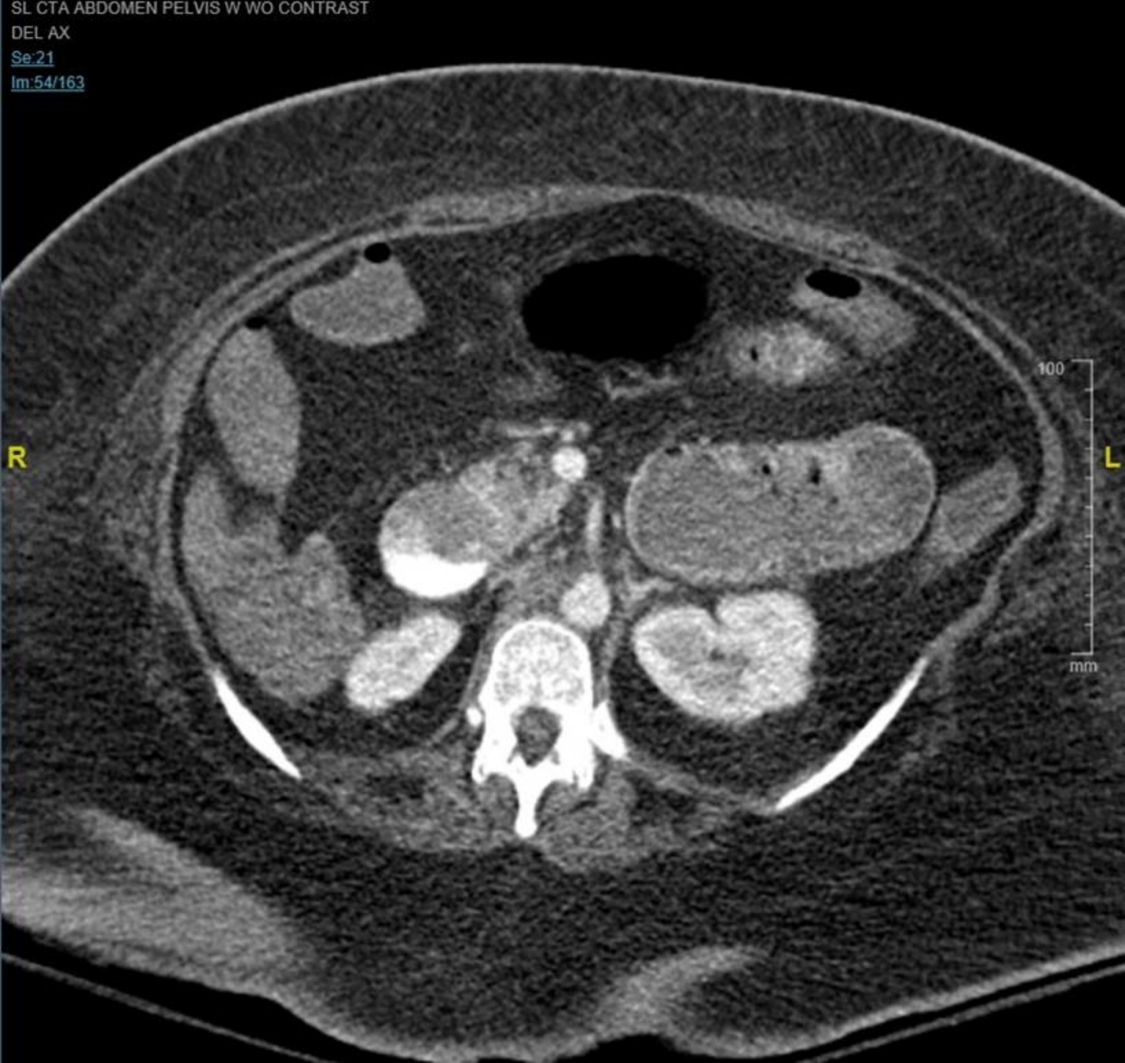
CT angiography abdomen and pelvis with contrast. Contrast extravasation within the duodenum compatible with gastrointestinal bleed.
